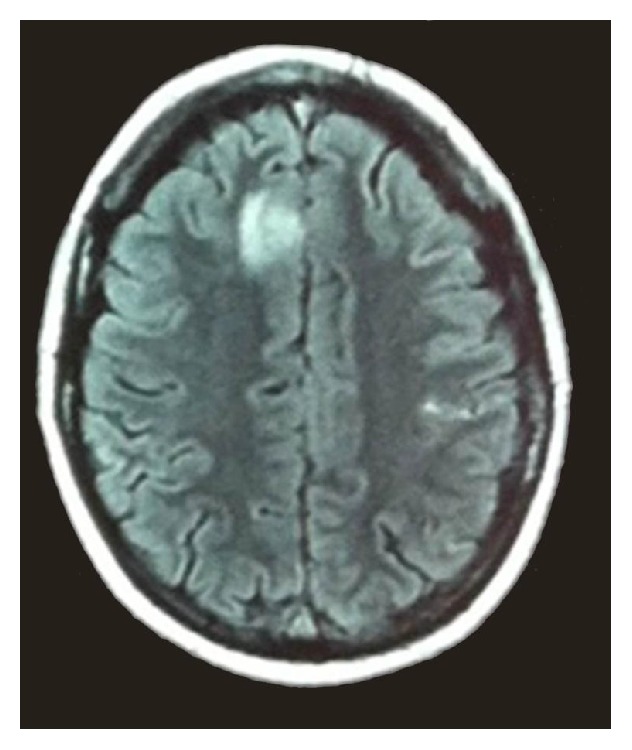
Axial FLAIR MRI shows peripheral hyperintensity in the right frontal lobe as well as abnormal high signal intensity involving the superior sagittal sinus, findings suggestive of cerebral venous thrombosis.
